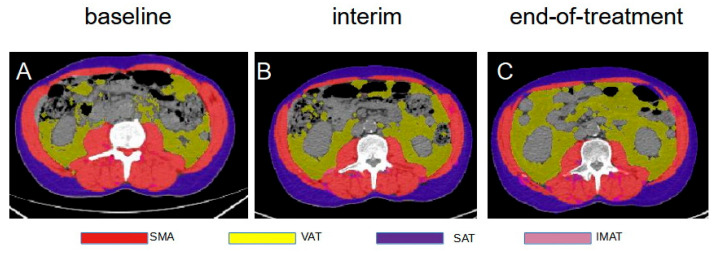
Serial axial CT images of a patient at baseline, interim and end-of-treatment [^18^F]FDG PET/CT with SMA (red), VAT (yellow), SAT (blue) and IMAT (violet) measurements. (A) axial image of a baseline scan; (B) axial image at the same slice of the interim scan; (C) axial image of the same slice of end of treatment scan.
